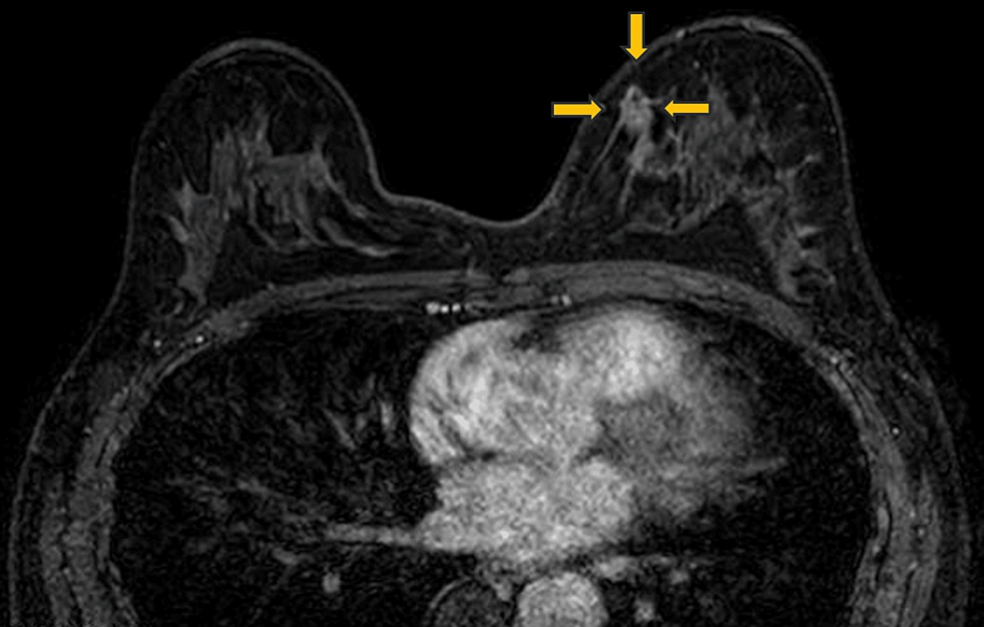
Dynamic contrast-enhanced MRI image showing a 10 mm mass with a slow-persistent enhancement pattern (type I curves). MRI, magnetic resonance imaging
